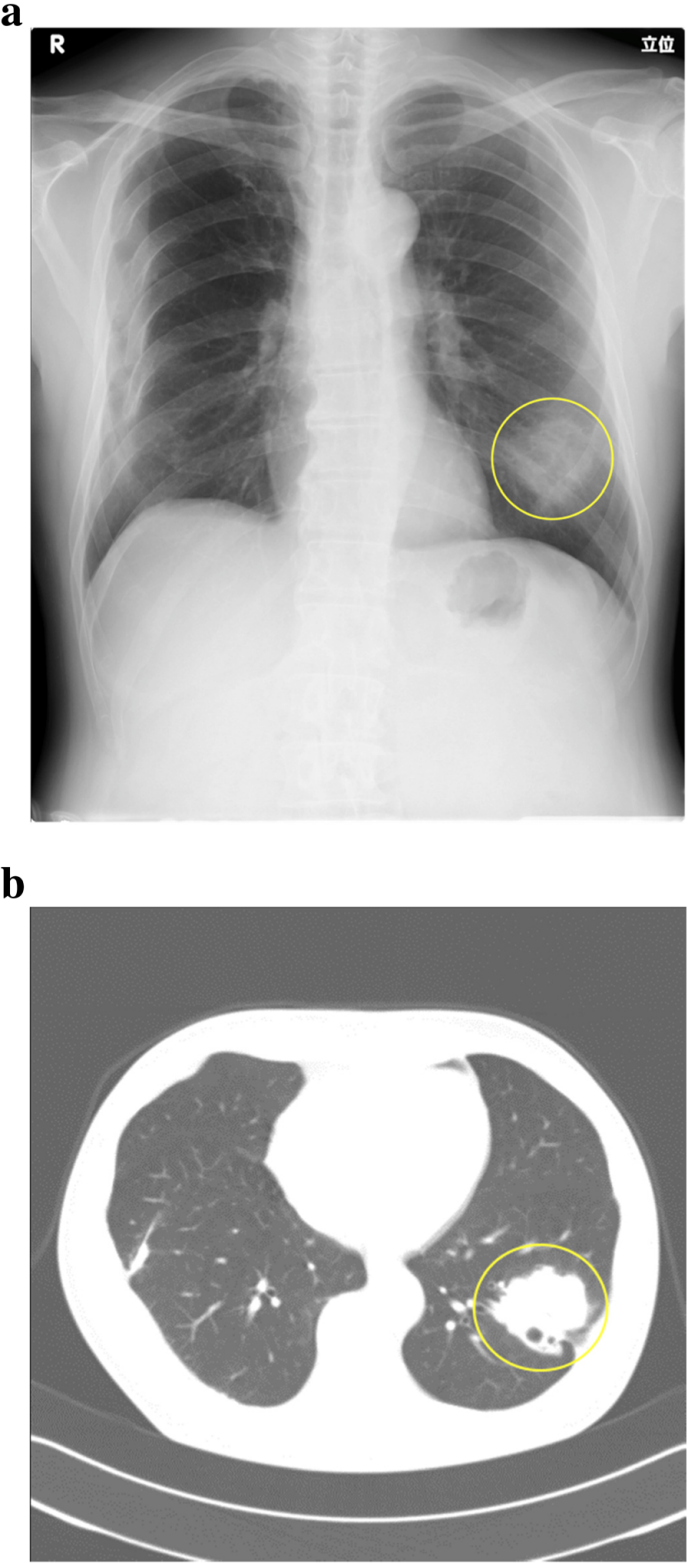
a Chest X-ray shows a 50-mm mass in the left lower field (yellow circle). b Chest CT scan shows a 47-mm tumor in the left lower lobe lateral basal segment (yellow circle)
